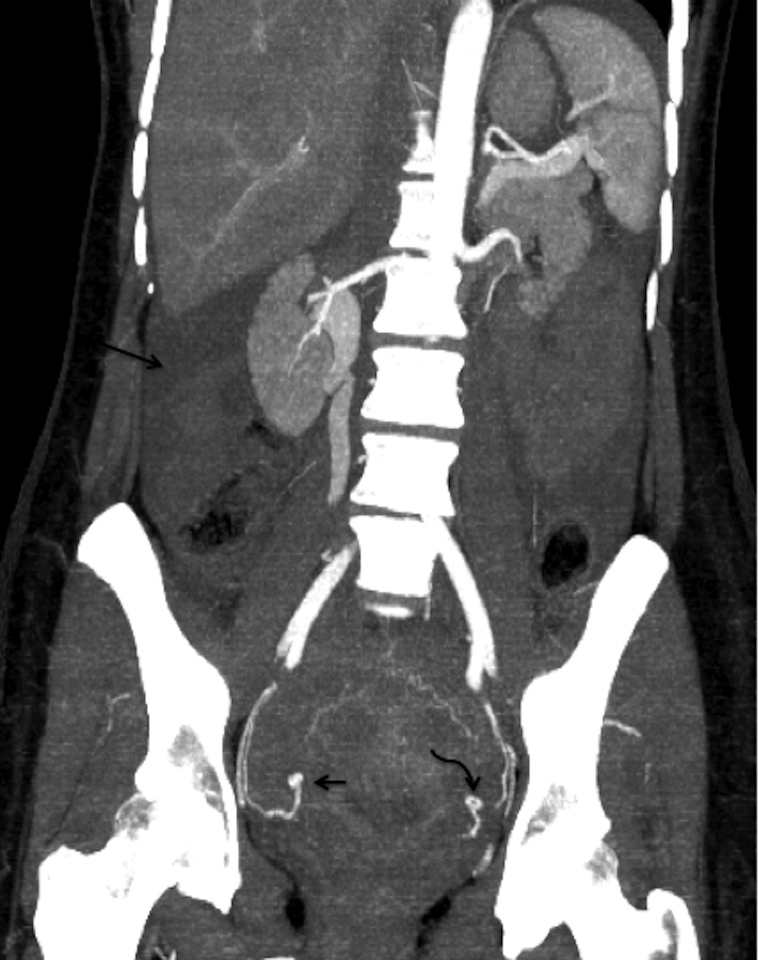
Coronal maximum intensity projection reconstructed images of the CT angiogram clearly demonstrates the pseudoaneurysm of the ascending portion of the right uterine artery (short arrow) compared with normal appearance of the left uterine artery (curved arrow). Long arrow indicates free fluid in the abdomen on the right side.
